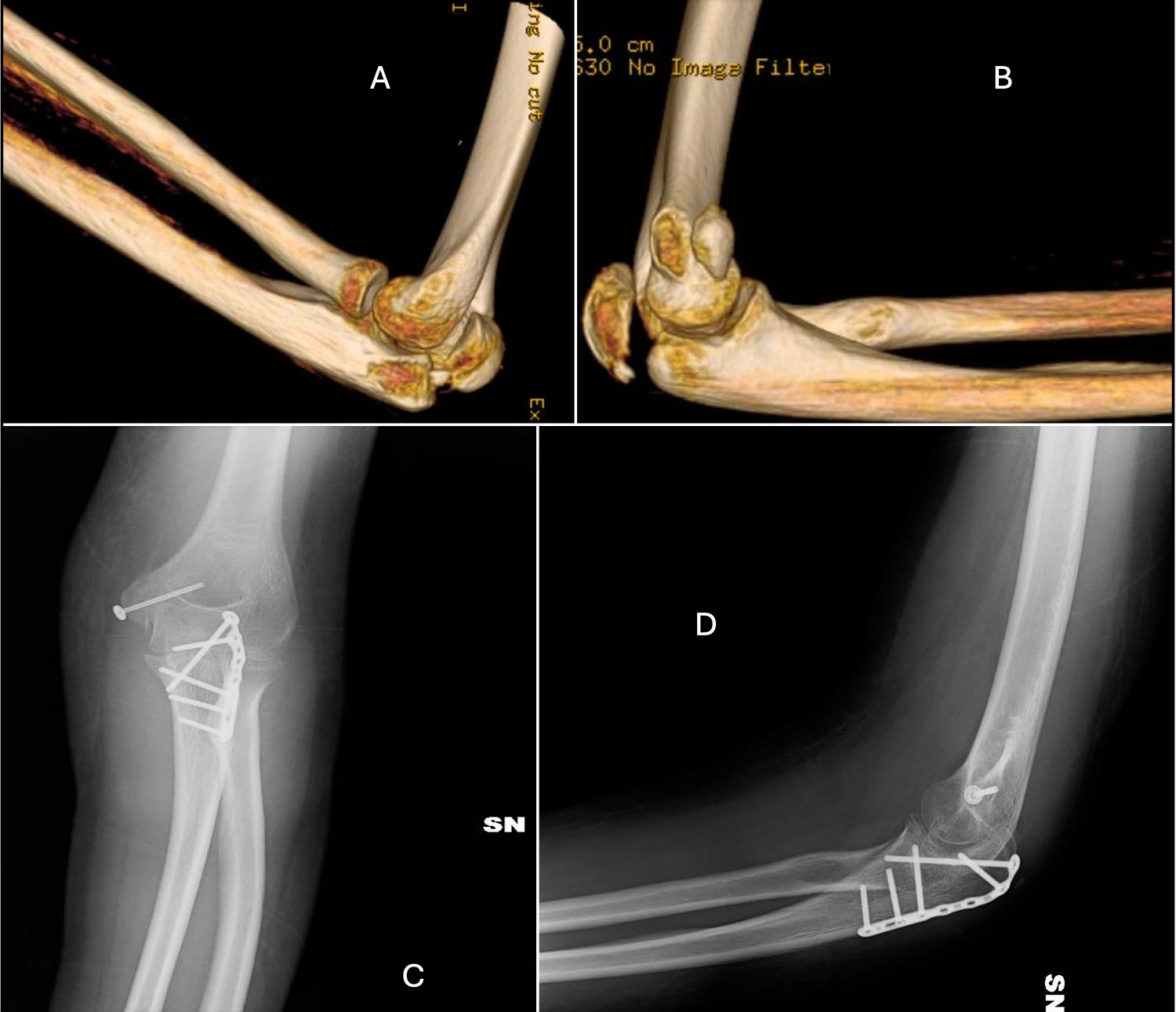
Images A and B display the 3D reconstruction of a complex elbow dislocation with fracture of the olecranon and epitrochlea. Images C and D show postoperative anteroposterior (AP) and lateral X-rays, where the olecranon plate and a screw with a washer for epitrochlear fixation are visible
